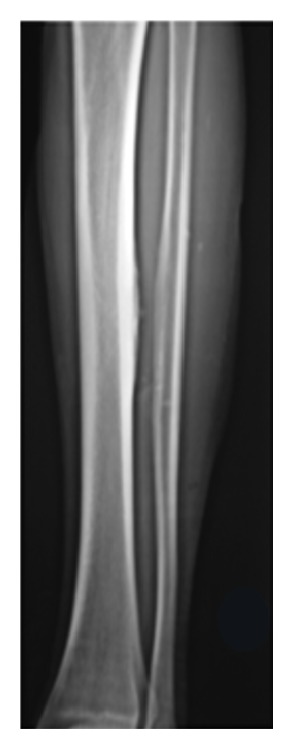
Postoperative anteroposterior radiograph showing complete excision of the ossification.
